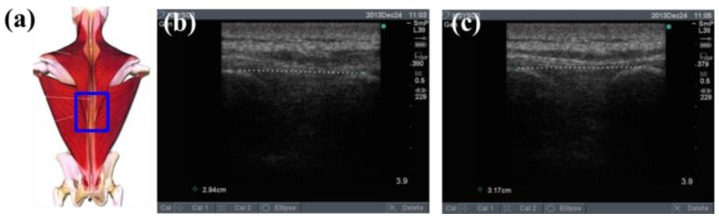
Results from ultrasound images: (a) measured mid erector spinae position; (b) preparatory action; (c) anteflexion action.
